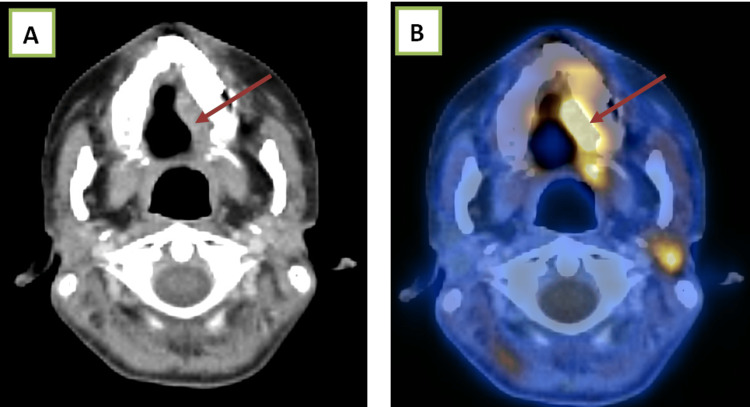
(A) Contrast-enhanced computed tomography and (B) fused PET-CT images show soft tissue thickening and heterogeneous enhancement along the left lateral border of the hard palate, with significant uptake on the fused PET-CT image. A heterogeneously enhancing left level II lymph node with significant Ga-FAPI uptake is also observed. Ga-FAPI, gallium fibroblast activation protein inhibitor; PET-CT, positron emission tomography-computed tomography
